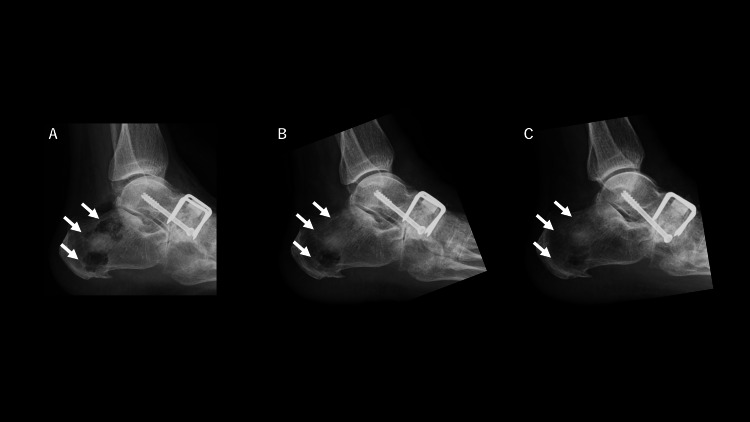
Postoperative radiograph of the foot (~14 days) (A) Just after surgery, Day 0: Talonavicular joint fusion is complete. The bony defect at the harvest site is supplemented with ReBOSSIS-J®. Three white arrows indicate the site of calcaneal bone harvesting. The central part was especially filled with ReBOSSIS-J®. The superior and inferior parts seemed to be less filled with material. (B) After one week, material resorption and new bone formation had already started and progressed two weeks after surgery (C). Three white arrows indicate the site of calcaneal bone harvesting. Not only the central part but also the superior and inferior parts were also filled with the newly formed bone.
